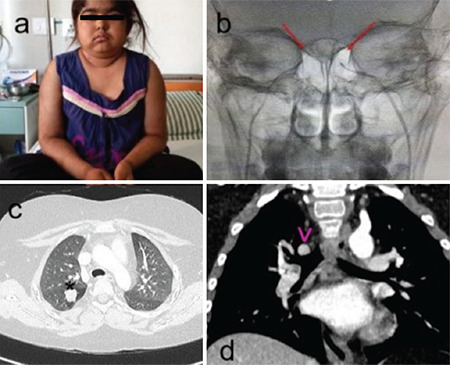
(a) Pre-operative photograph of patient showing cushingoid facies, hirsutism and obesity, (b) bilateral inferior petrosal sinus sampling with red arrows indicating the microcatheters in petrosal sinuses, (c) axial and, (d) coronal view of computed tomography chest showing a smoothly margined nodule of 15 mm diameter in the apical segment of the right upper lobe
